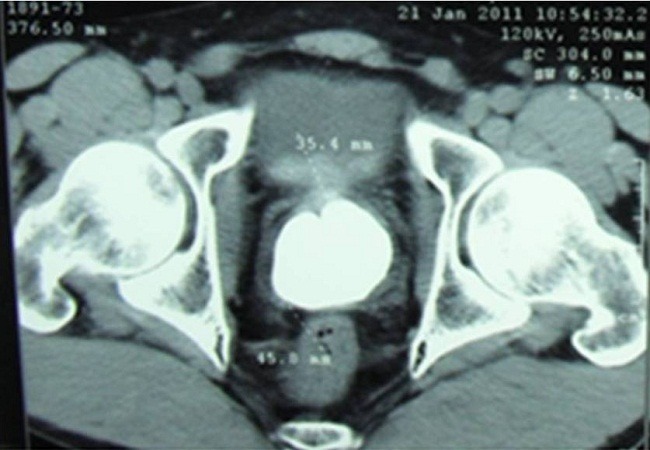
Nonenhanced CT Scan showing replacement of prostate gland with calculi
